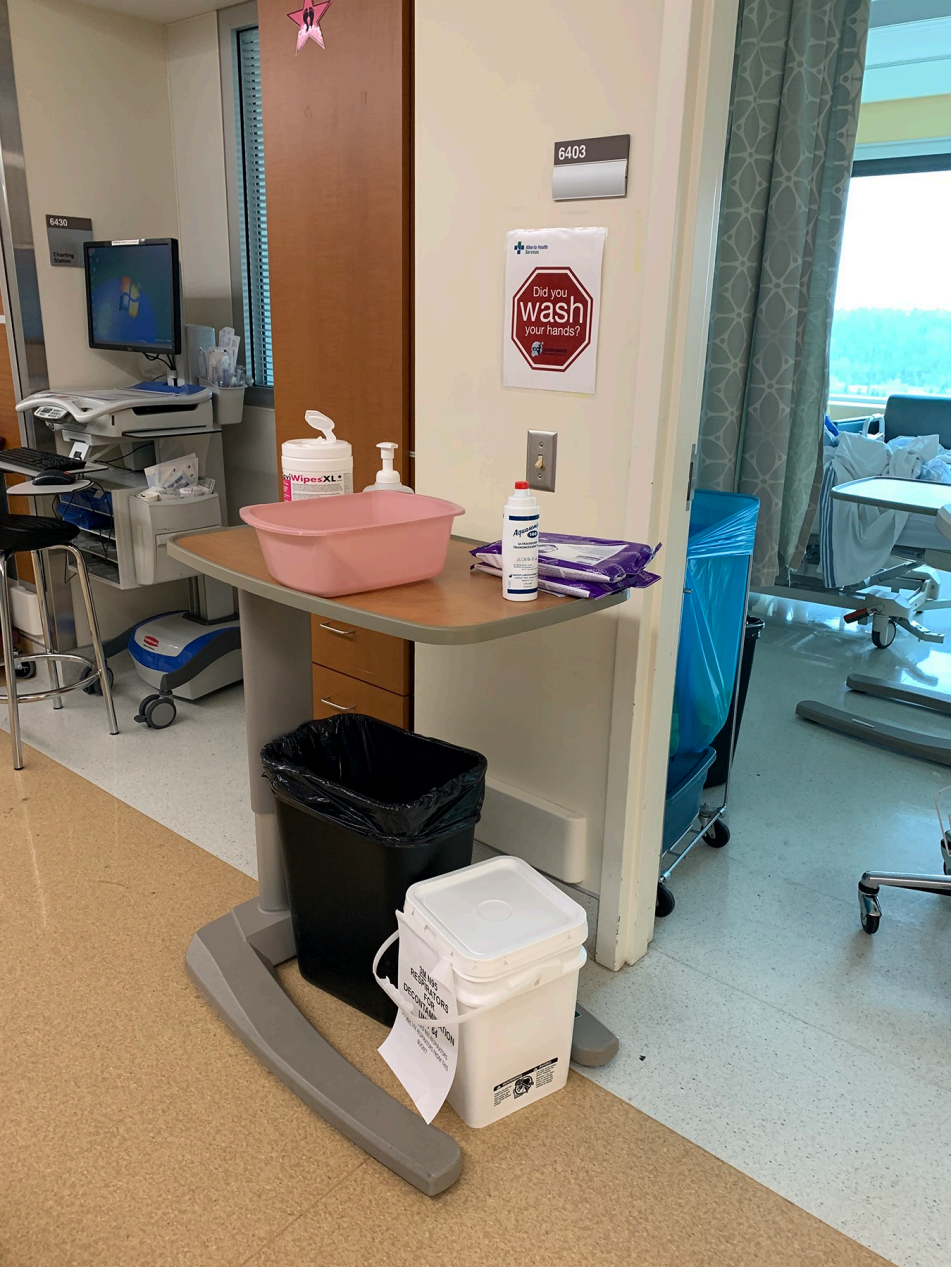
HF/ethnography team members observed clean supplies (pictured here: Sterile wipes and ultrasound gel) being placed on contaminated tray tables outside patients’ rooms.
